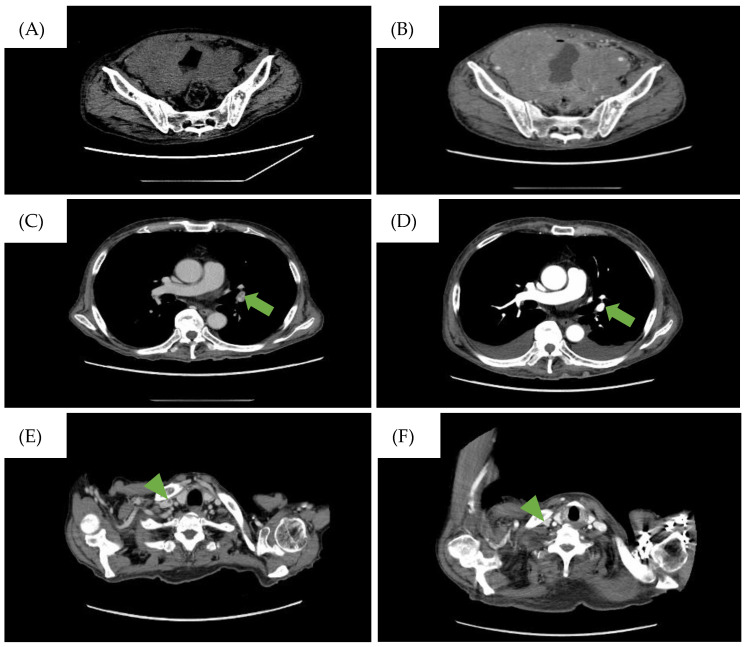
CT findings during the clinical course of the patient. (A) CT scan on the day the patient was referred to our hospital. Diffuse bladder wall thickening up to 7 mm was observed. (B) CT scan taken when the patient complained of abdominal pressure. A large intra-abdominal mass was observed. The low-density area represents the pulmonary embolism (PE) in the (C) left pulmonary artery and (E) the right internal carotid artery and disappearance of the PE after treatment in the (D) left pulmonary artery (→) and (F) the right internal carotid artery (▶︎).
